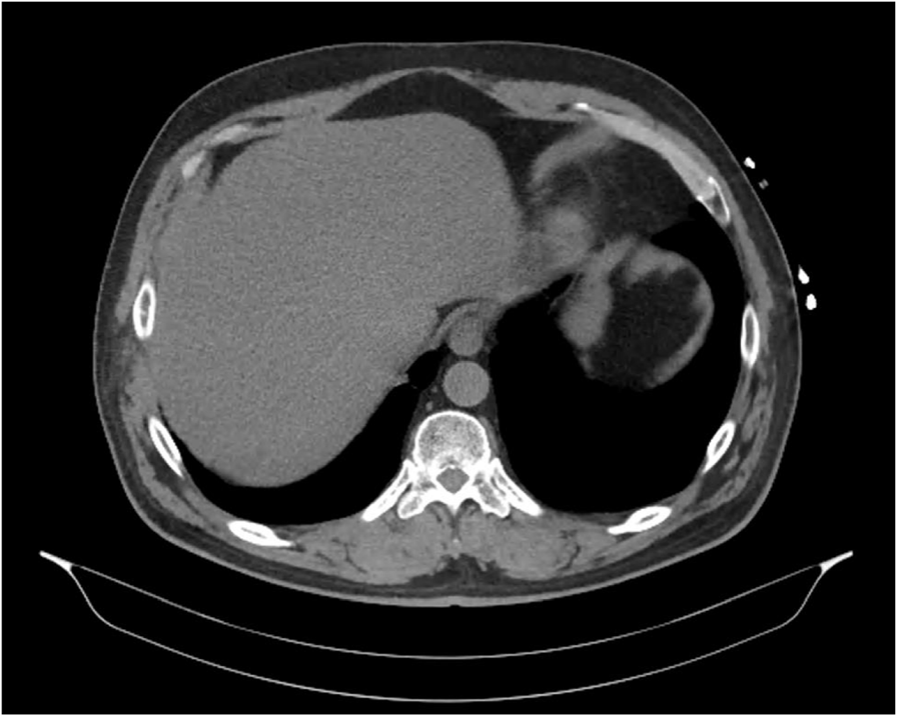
Computed tomography (CT) of the abdomen and pelvis in a patient with suspected gastroenteritis. Image was obtained within 24 hours of presentation to the hospital. CT demonstrated cholelithiasis but no other abnormality
